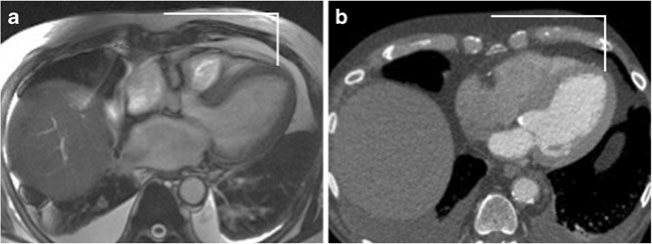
Assessment of left ventricle apex on MRI. Location of the apex in the intercostal space and the distance of this access site from the midline can be measured and marked using axial non-gated SSFP sequence (a). Same site location by CTA in this patient (b)
